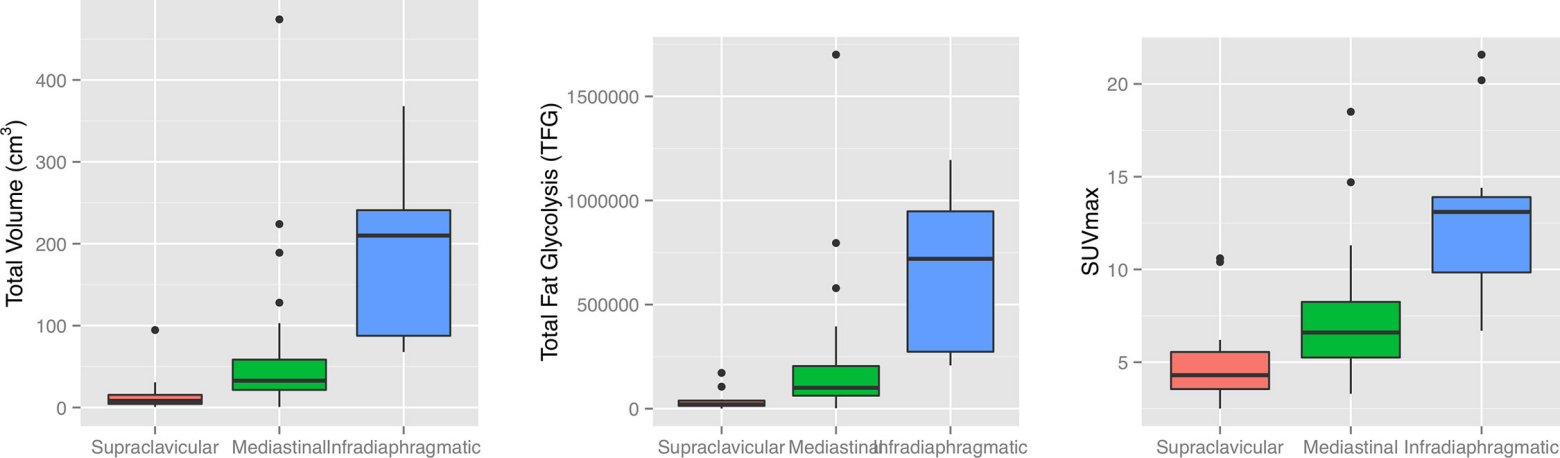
Quantification of glycolysis in the different depots. A clear increase of all three metabolic PET-parameters MFV, TFG and SUV_max_, from the supraclavicular depots to the more caudally located infradiaphragmatic depots can be appreciated.
